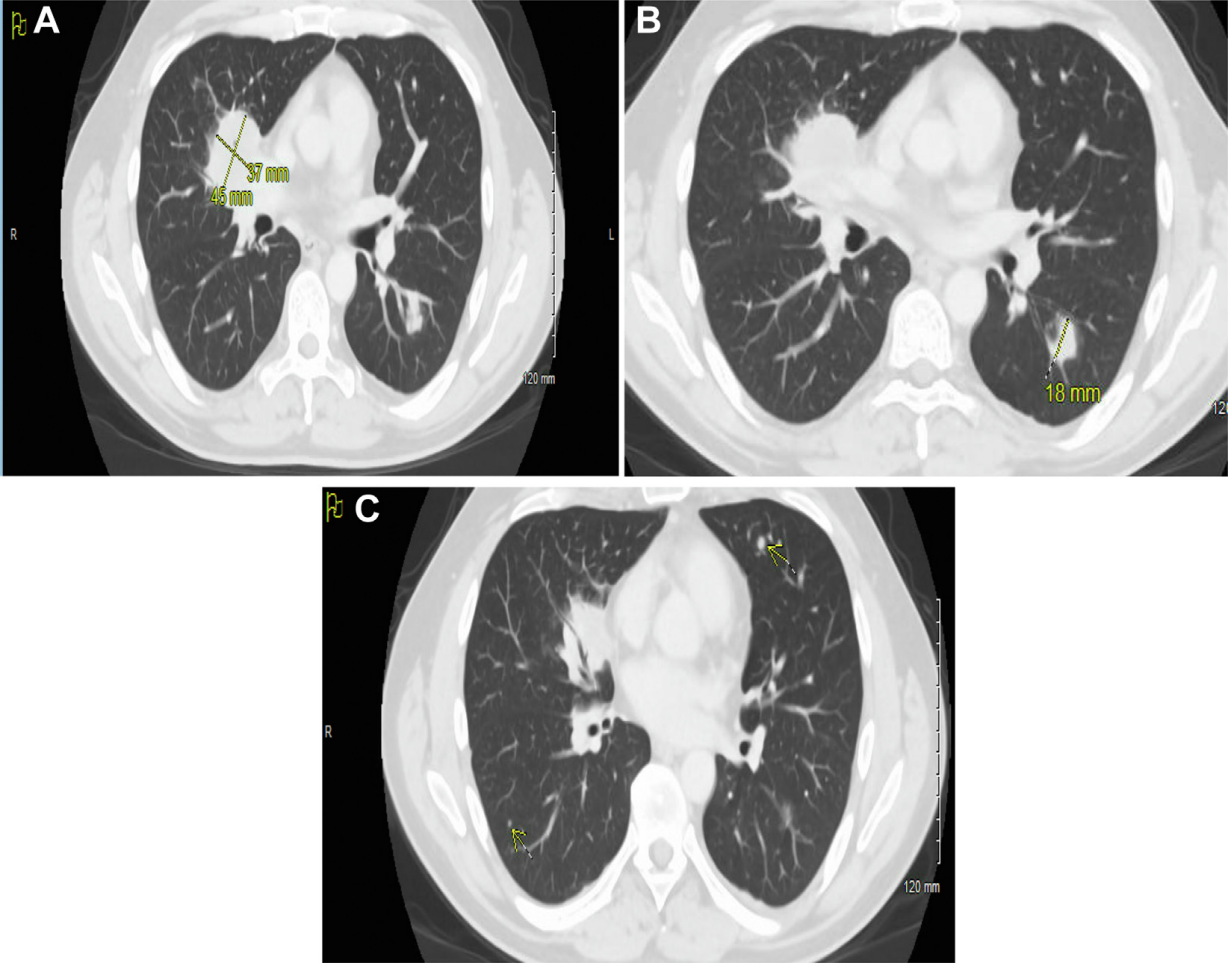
Imaging of Lung Nodules: Computed Tomography Angiography of the Chest (A) An irregular heterogenous mass anteriorly in the right hilum extending into the right upper and middle lobes and measuring 4.5 cm × 3.7 cm. (B) An ill-defined nodule measuring up to 18 mm in the left lower lobe. (C) Arrow on right side of image indicating a tiny 2-mm nodule medially in left lower lobe. Arrow on the left side of image indicating another tiny approximately 2mm rounded nodule in right lower lobe.
